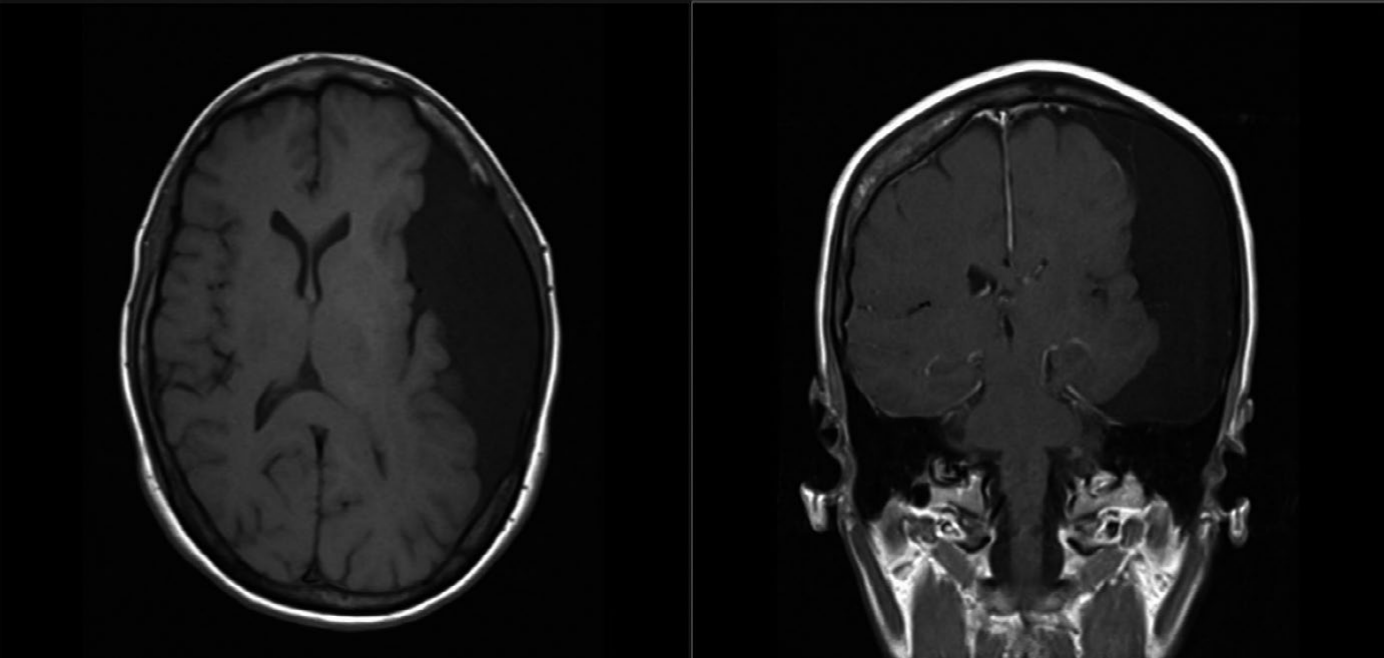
Magnetic resonance images of the head in axial (left) and coronal views (right)—showing large left‐sided extra‐axial collection with some internal septations (without postcontrast enhancement), that exerts mass effect on the adjacent left cerebral hemisphere and a rightward midline shift of about 3 mm
